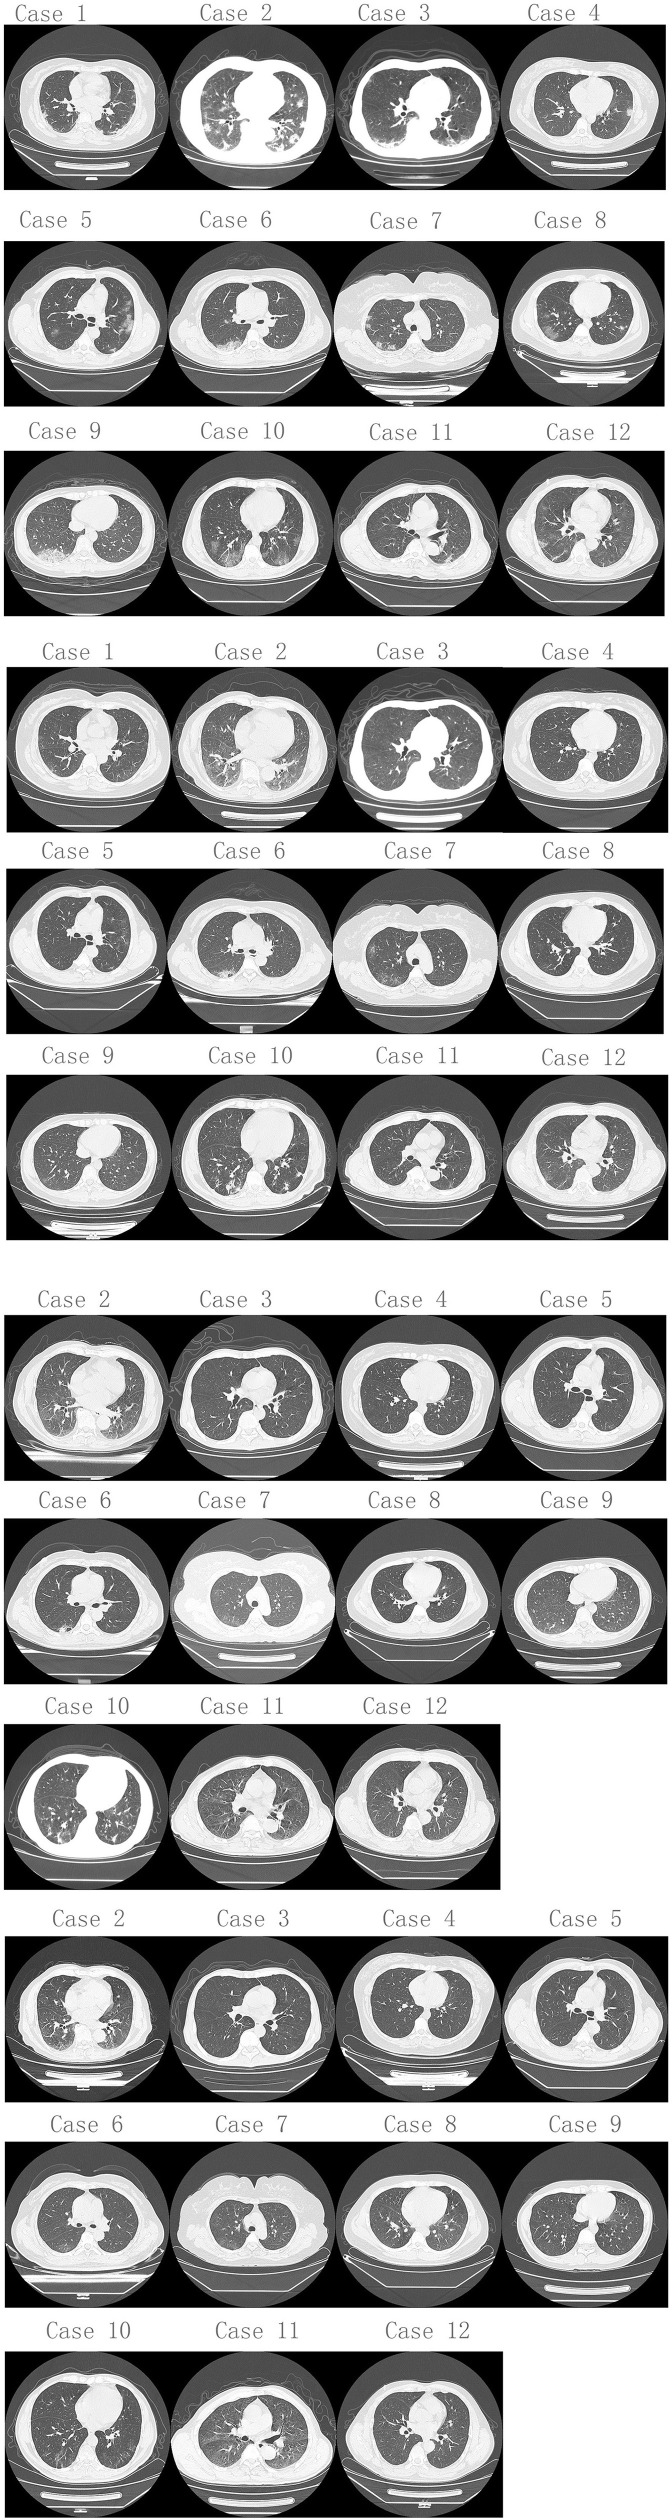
Chest CT changes from 1st to 2nd hospitalization of 12 re-admitted patients with COVID-19. (A) Chest CT of 1st hospitalization. Twelve patients (100%) had infectious lung lesions, mainly ground glass lesions, and both lungs were involved to varying degrees. (B) Chest CT of 12 re-admitted patients when 2nd hospitalized. Five patients (41.7%) still had lung infectious lesions, and only 1 patient (8.3%) had progressive lung infectious lesions compared to discharge before. The rests had much better smaller infectious lesions than discharged from hospital. (C,D) Chest CT changes during 2nd hospitalization of 11 re-admitted patients (except case 1).
